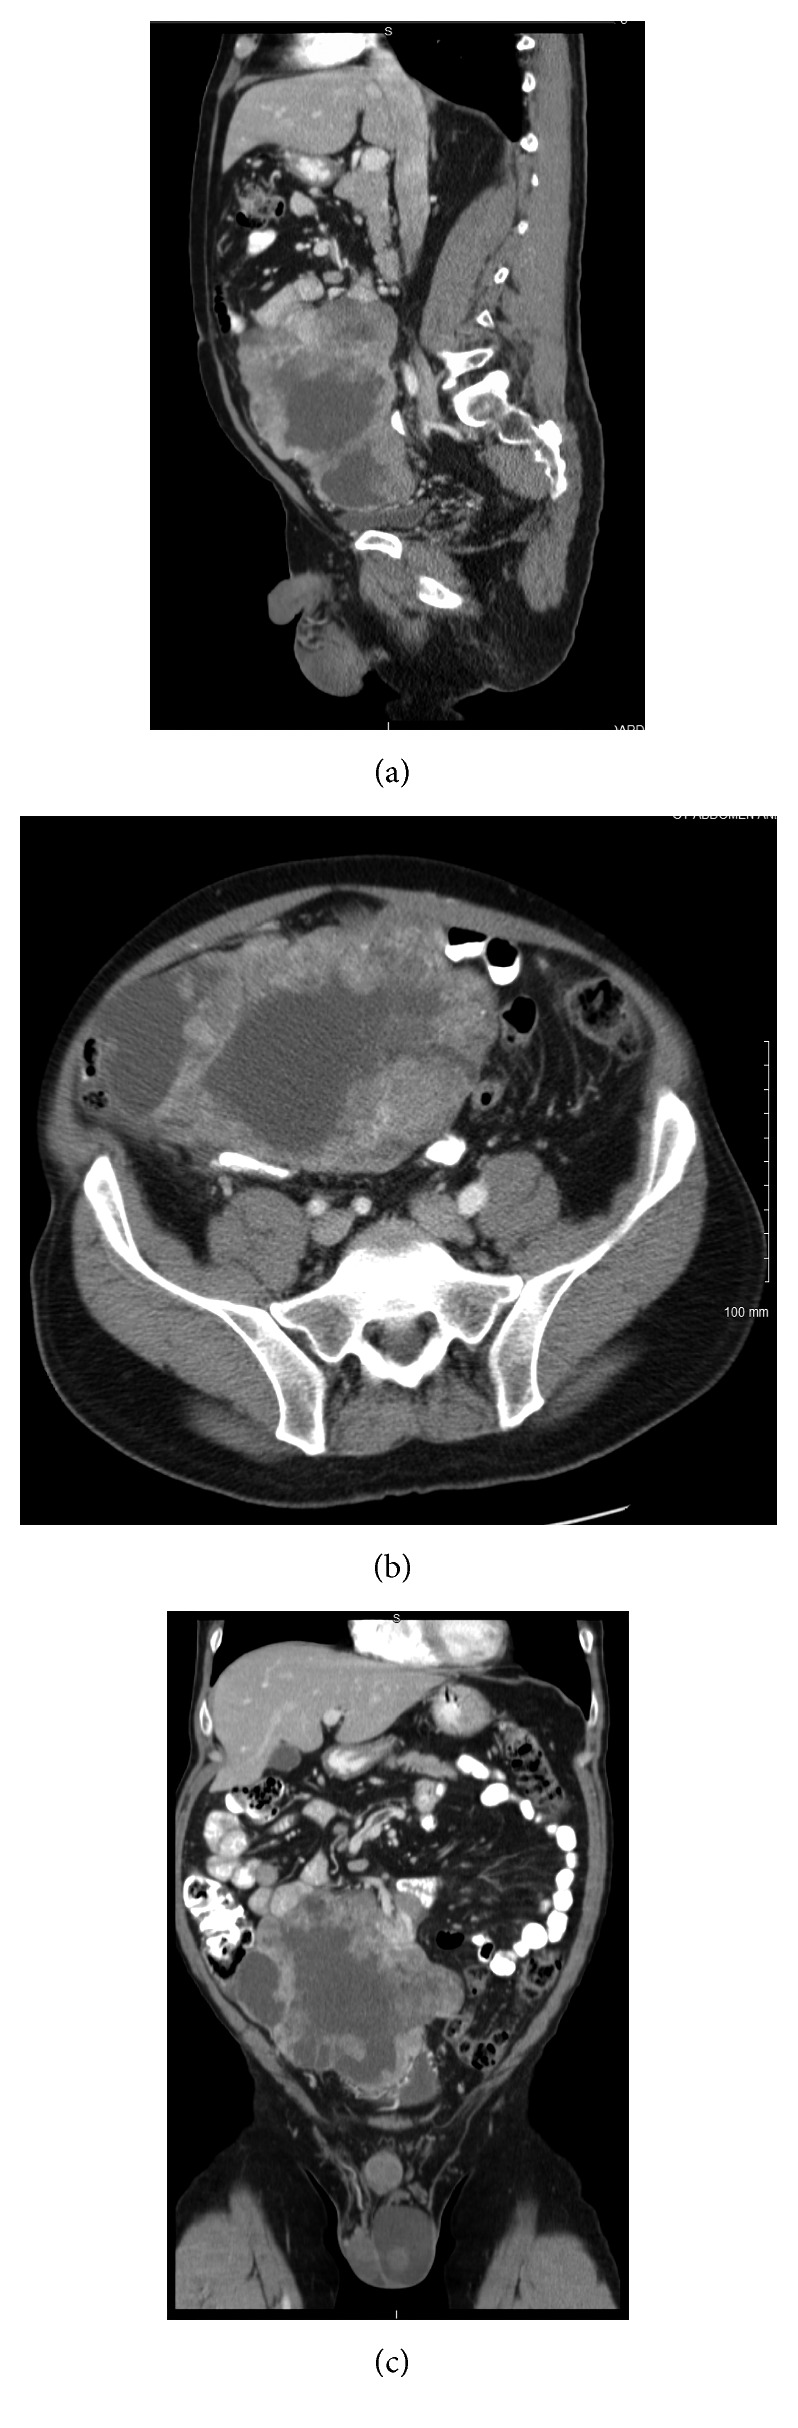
Sagittal (a), axial (b), and coronal (c) images of CT with IV contrast showing the large, lobulated primary mass that was discovered in January 2013.
